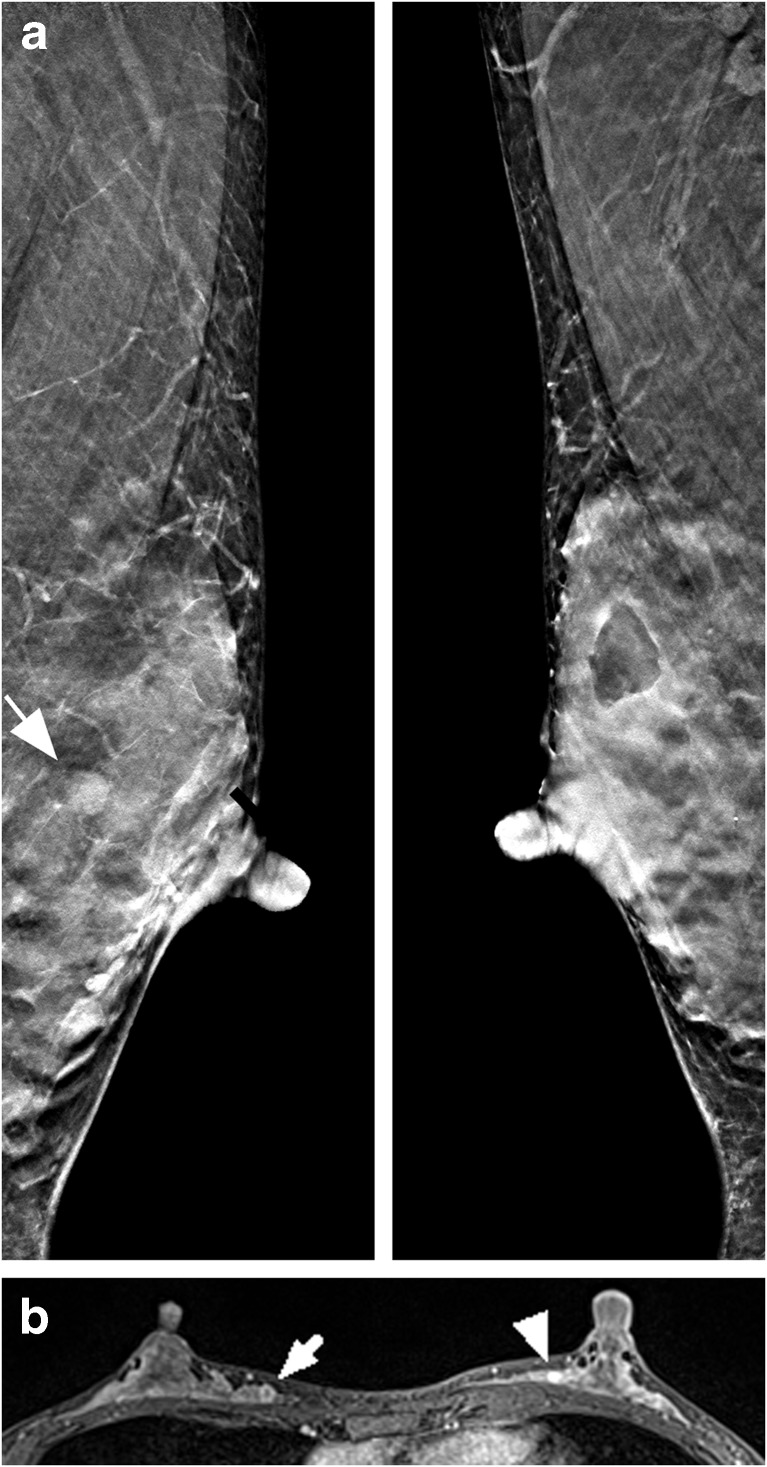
A 37-year-old woman with a 15-mm mucinous carcinoma in the right breast and a benign fibroadenoma in the left breast. a Bilateral DBT slices in the mediolateral oblique projection with the fibroadenoma highlighted (*arrow*) in the left breast. Both readers reported breast composition d and percent density was estimated to 70% by Libra. The carcinoma was missed (BI-RADS 1) by both readers and the fibroadenoma was rated BI-RADS 4 by reader 1 and BI-RADS 3 by reader 2. b Axial post-contrast T1-weighted MRI with the carcinoma highlighted in the right breast (*arrow*) and the fibroadenoma (*arrowhead*) in the left breast. The amount of fibroglandular tissue was reported heterogeneous and the background parenchymal enhancement was marked. The final report stated BI-RADS 3 finding in the right breast and BI-RADS 4 finding in the left breast
